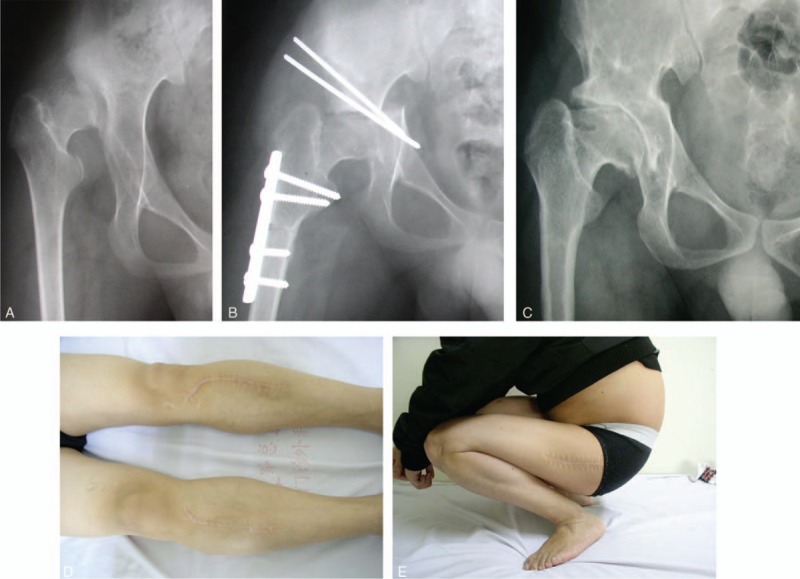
(A) Fifteen-year-old boy. Preoperative hip radiograph showing luxation in right hip. Defects exhibiting in the anterosuperior femoral head. (B) Hip radiograph taken immediately after the periosteal transplantation combined with surgical reduction of the femoral head. (C) Hip radiograph taken 19.3 years after the surgery showing good congruency between the femoral head and the shell and the both joint space well preserved. (D) Photo taken postoperatively showing good wound healing after cutting autologous tibial periosteal. (E) Photo taken 19.3 years after the surgery showing excellent hip range of motion.
